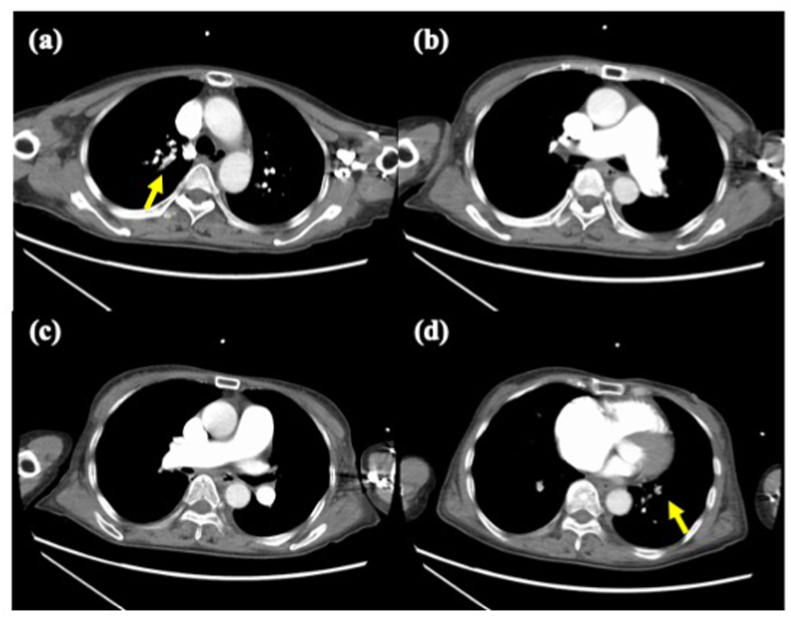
The enhanced CT showed a small defect of fresh thrombus (yellow arrows) in the bilateral pulmonary arteries at the segmental or subsegmental levels and signs of CTEPH. (For interpretation of the references to colour in this figure legend, the reader is referred to the Web version of this article.)
